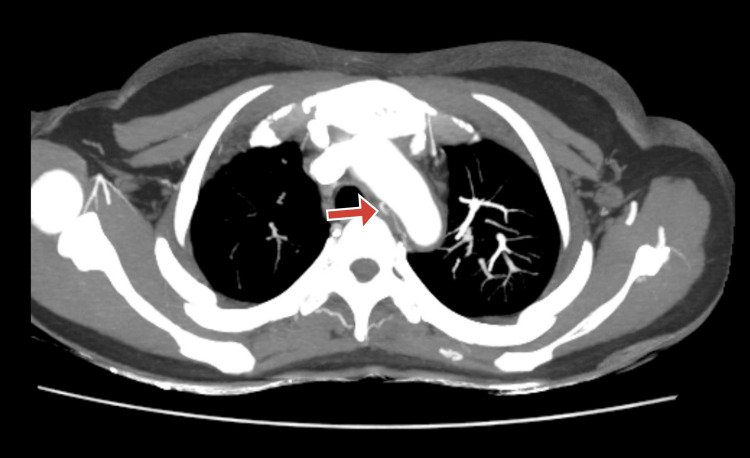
CT aortogram with an arrow showing the thickening of the wall of the arch of the aorta
